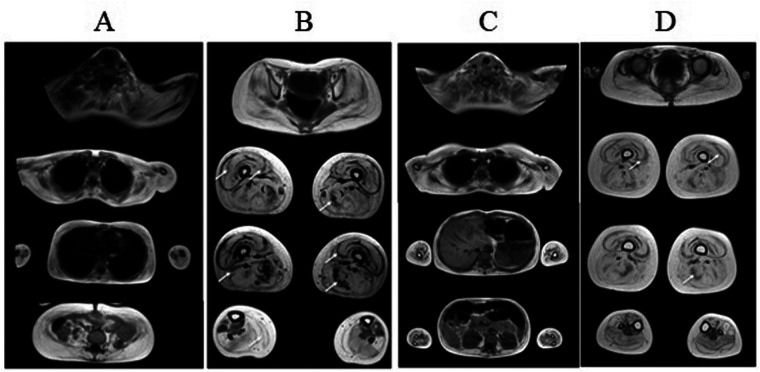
Whole body muscle MRI of proband (A–B) and proband's sister (C–D). Axial T1-weighted imaging revealed similar patterns of fatty infiltration and muscle involvement in both children. The third and forth grade of fatty muscle infiltration in upper and lower limb muscles are noted. The cores of preserved muscle within the vastus lateralis (arrows), vastus medialis in both children (“sandwich”-like pattern), relative preservation of the semimembranosus, adductor magnus muscles (arrows) and tibialis posterior, extensor digitorum longus muscles.
